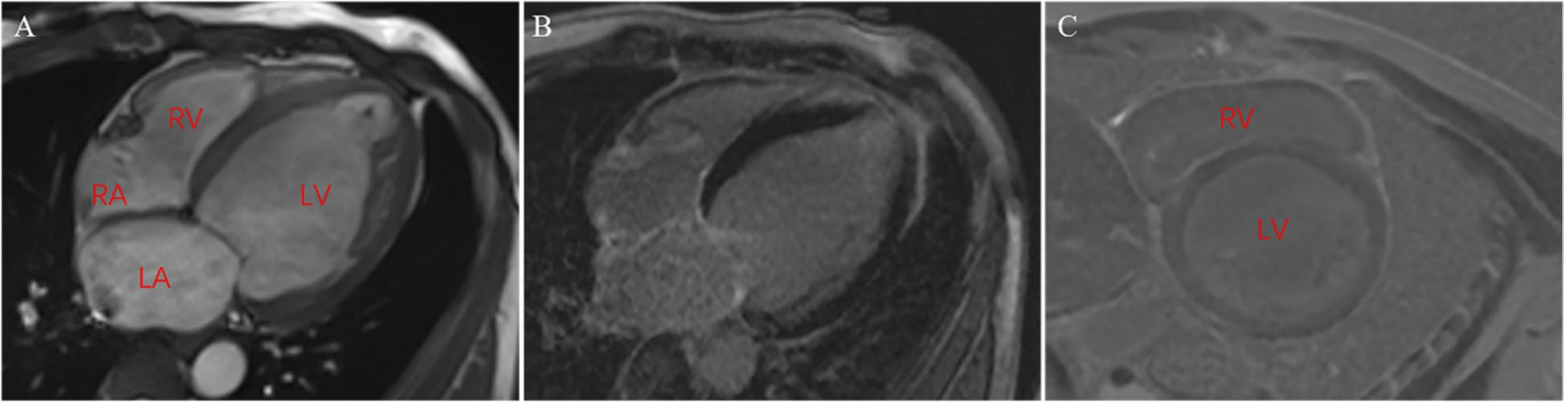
Cardiac MRI. A Four-chamber view showing marked LA and LV dilatation (LA: 41 mm; LV end-diastolic diameter: 78 mm; EDV: 361 ml) with highly reduced EF (EF 25%). B, C LGE image did not show significant delayed enhancement. B Four-chamber view. C Short-axis view
